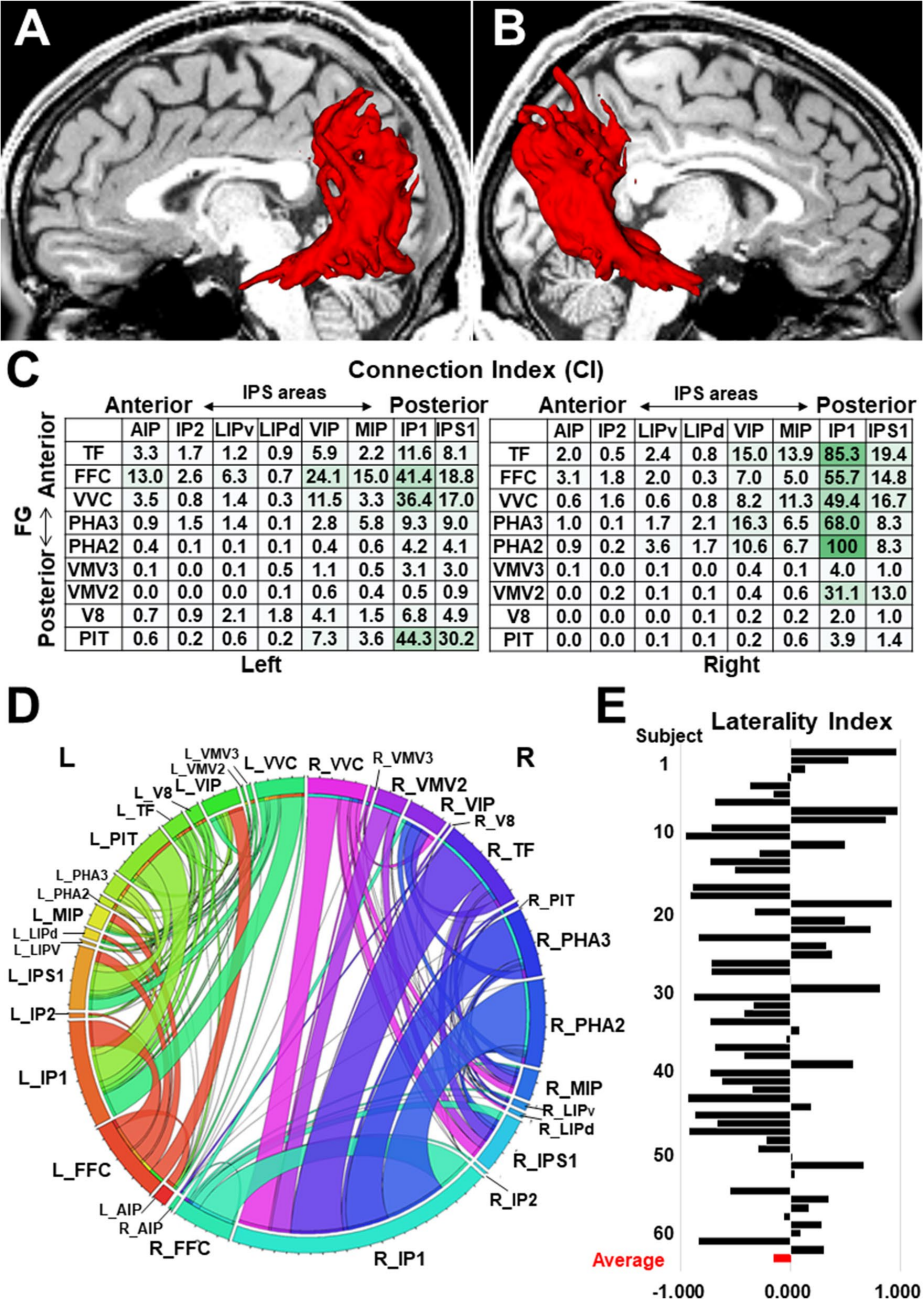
Streamline density maps, Connection index (CI), Connectogram, and Laterality index (LI). (A,B) Lateral view of streamline density maps for 60 subjects’ IPS-FG tractography, overlaid on T1-weighted image. (C) The tables indicate the Connection index (CI) for the tract numbers of each connection to link IPS areas and FG. Left; left hemisphere. Right; right hemisphere. Darker colors indicate the strength of connection. The data is average of healthy 60 subjects. (D) Connectogram representing bilateral connectivity patterns of IPS-FG, reflecting tract numbers of each connection. Circular color map represents the segmented brain regions that each streamline interconnects. The segmented arc and ribbon size represent the computed degrees of connectivity (the number of tracts) between segmented brain regions. The data is average of 60 subjects. (E) Graph indicates the laterality index (LI) for the tract numbers of IPS-FG in each subject (#1-60). The lowermost lane (red) is the average value of 60 subjects. The *laterality index* (left − right)/(left + right) shows the cerebral asymmetry. *AIP* anterior intraparietal, *VIP* ventral intraparietal, *MIP* medial intraparietal, *IP1, IP2* intraparietal 1, 2, *IPS1* intraparietal sulcus 1, *LIPv* lateral intraparietal ventral, *LIPd* lateral intraparietal dorsal, *PHA2, 3* parahippocampal area 2, 3, *V8* visual area 8, *PIT* posterior inferior temporal, *FFC* fusiform face complex, *VVC* ventral visual complex, *VMV2, 3* ventro-medial visual areas 2, 3.
